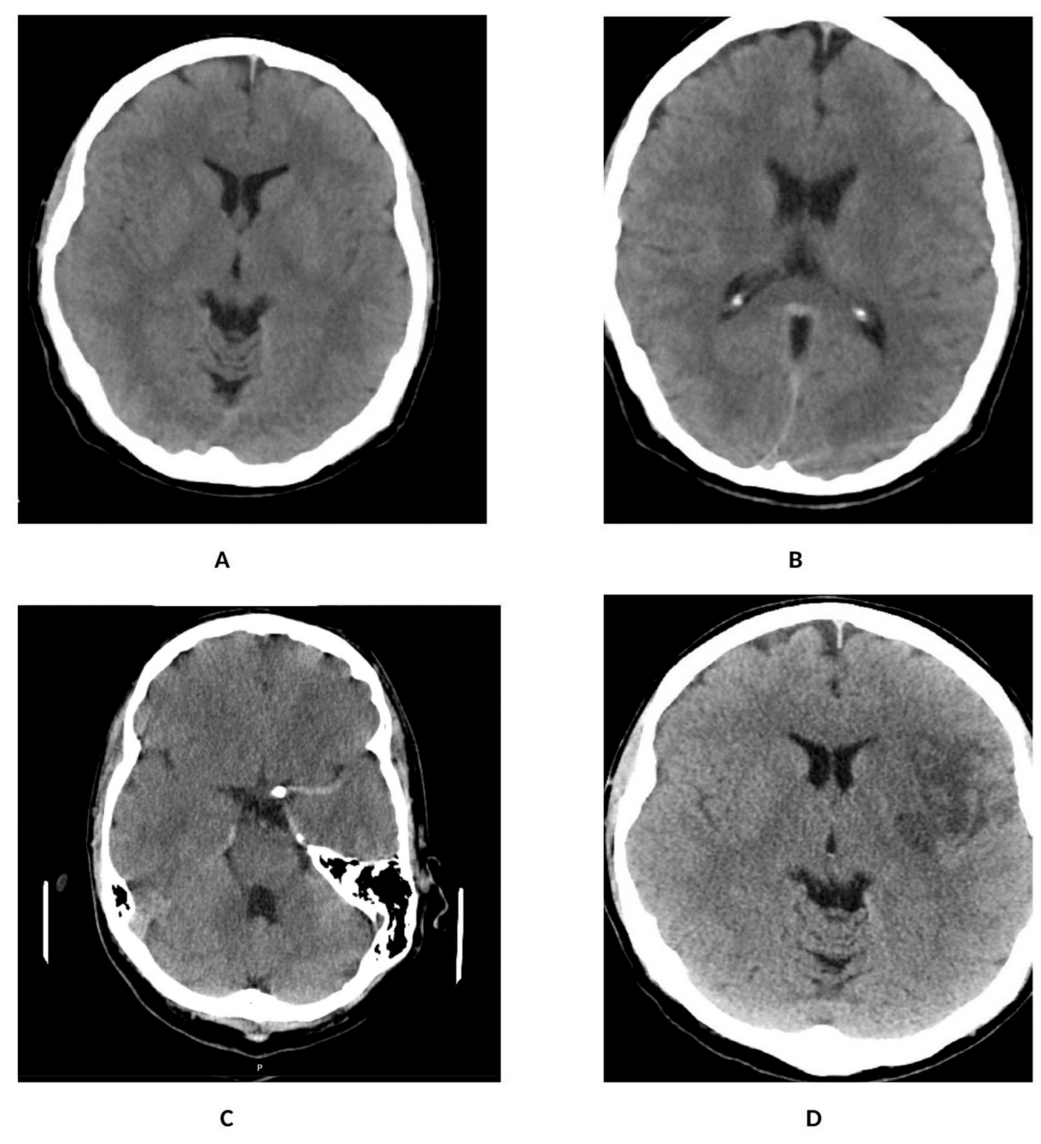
A: CT scan of the head on admission showing no acute findings. B: Second CT scan after the initial weakness resolved, showing no definite intracranial pathology. C: Evidence of a dense left MCA sign with corresponding occlusion of the left MCA (M1 segment). D: CT scan of the head post-thrombectomy showing an established infarct in the left MCA territory CT: computerized tomography; MCA: middle cerebral artery
